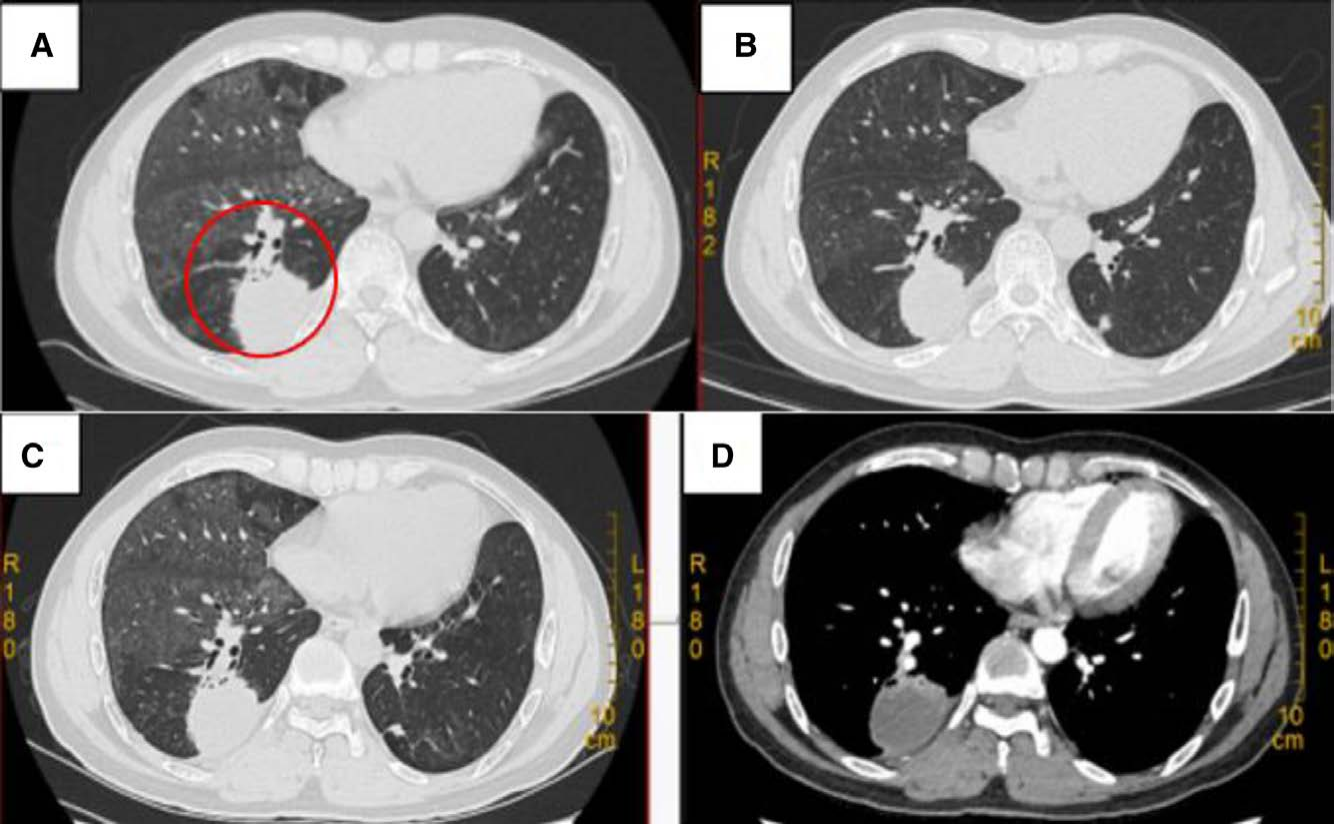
Infection-related lung CT. (A) Chest CT scan on April 18, 2024: new patchy opacity in the lower lobe of the right lung (red circle). (B) Follow-up chest CT scan on May 30, 2024 (compared with April 18, 2024, the ground-glass opacities in both lungs have improved). (C and D) Chest CT scan on April 22, 2024. CT = computed tomography.
